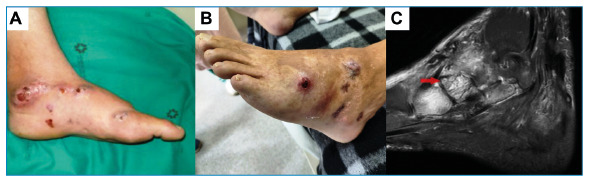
Clinical features; (A) Nodular lesions on left foot compatible with mycetoma; (B) Recurrence of symptoms after several years of medical treatment; (C) Magnetic resonance imaging (MRI) of left foot, revealing high-signal areas on fat-saturated T2 weighted sequence in the subcutaneous tissue and midfoot bones. Red arrow indicates tiny hypointense foci within hyperintense spherical lesions, known as the “dot-in-circle” sign.
